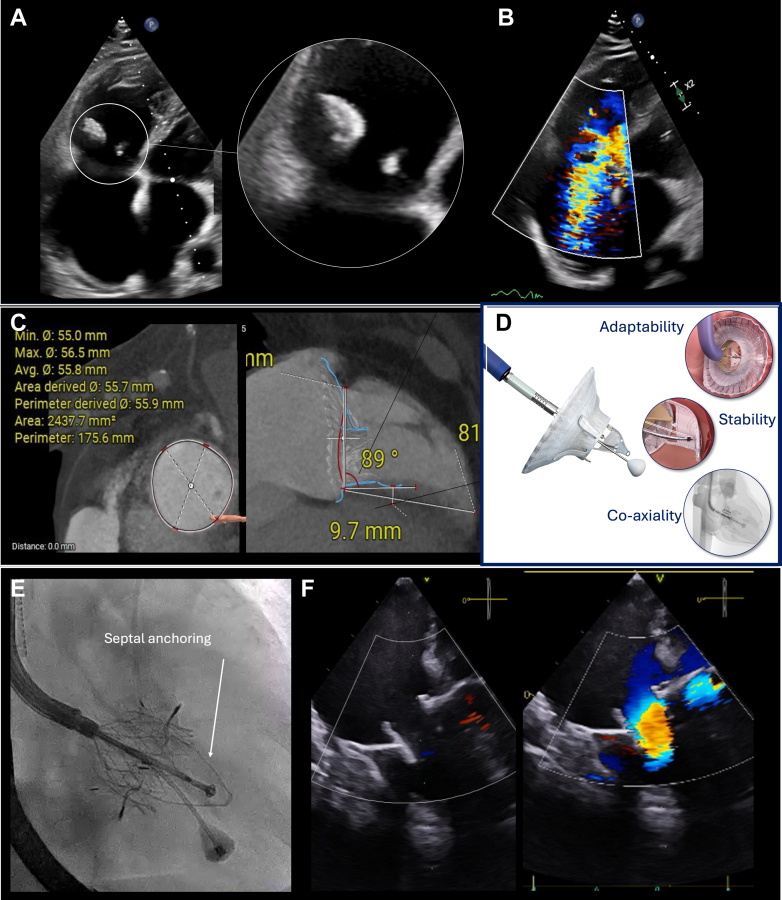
Baseline Echocardiography and CT Scan, Main Lux-Valve Plus Features, Fluroscopic View During Implantation and Final Echocardigraphic Result (A and B) Baseline transthoracic echocardiography (TTE) showing aseptic vegetations (left, white circle) and torrential tricuspid regurgitation (TR) (right). (C) Computed tomography (CT) scan assessing anatomical suitability for transcatheter tricuspid valve replacement (TTVR). (D) The LuX-Valve plus system (Jenscare) highlighting key features: coaxial alignment via jugular approach, stability through septal anchoring, and adaptability with a flexible design and large atrial skirt. (E) Fluoroscopic view demonstrating LuX-Valve system (Jenscare) deployment stabilized by the septal anchor (white arrow). (F) X-plane transesophageal echocardiography (TEE) confirming the final successful result.
